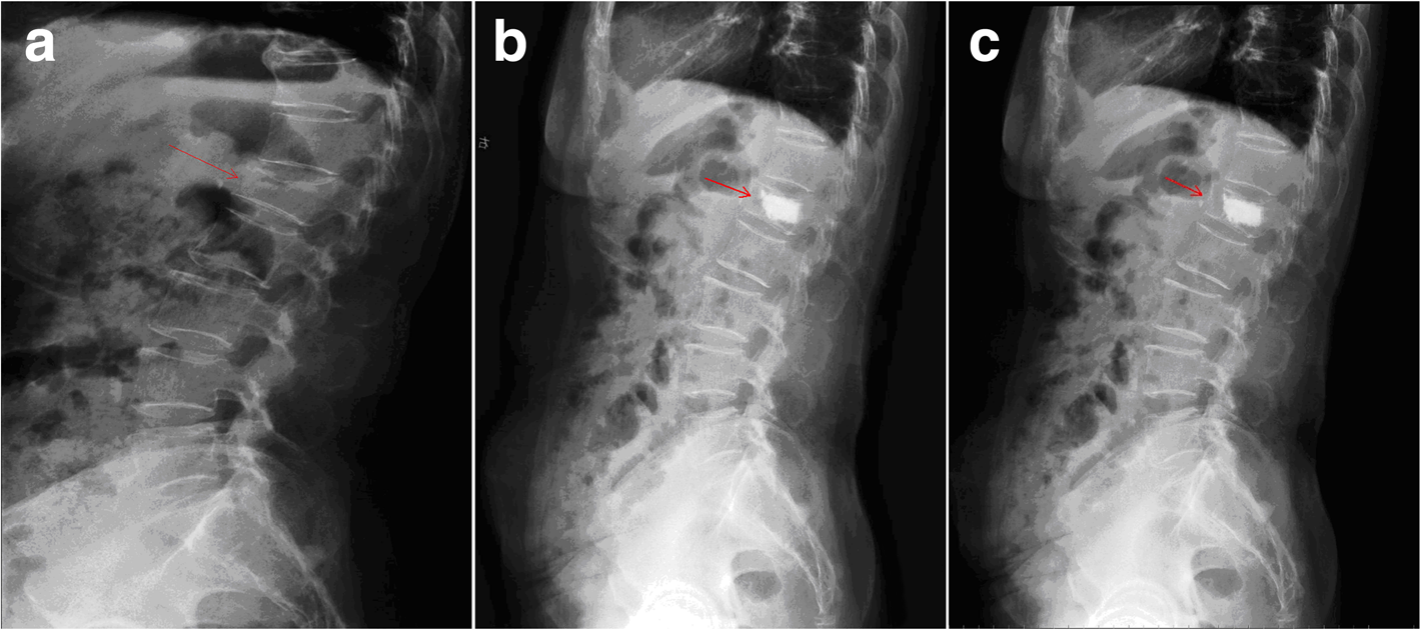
A patient with a BMD T-score. a Preoperative X-ray examination revealed vertebral compression fracture at L1; the anterior vertebral body height was 23.3 mm and compressed 17.6 % and the central vertebral body height was 17.5 mm and compressed 38.2 %. Cobb angle was 18.2°. b Roenterography 1 week post surgery revealed that the anterior vertebral body height was 27 mm and was restored to 84.4 % of the original height and the central vertebral body height was 25.0 mm and was restored to 88.2 % of the original height. Cobb angle was 15.9°. Bone cement filling was adequate. c Roenterography at the final follow-up (8 months post surgery) revealed that the anterior vertebral body height was 26.8 mm and was restored to 83.8 % of the original height and the central vertebral body height was 24.4 mm and was restored to 86.1 % of the original height. Cobb angle was 16°. Bone cement filling was adequate
